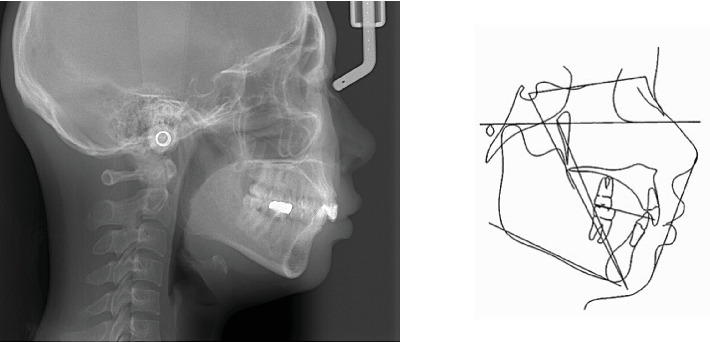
Pretreatment lateral cephalometric radiograph and tracing.
